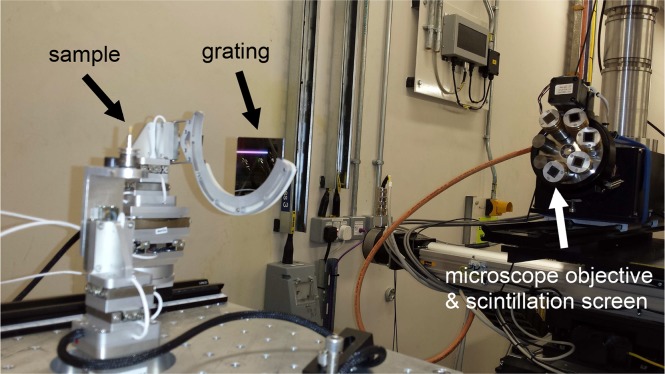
Experimental setup using a single phase grating (period: 10 *μ*m) mounted at a distance of 5 cm downstream of the sample. A high-resolution X-ray detector (effective pixel size: 1.13 *μ*m) is placed 72 cm further downstream to record the interference pattern created by the grating. The phase shift of the X-ray wavefront induced by the sample results in a distortion of the interference pattern.
